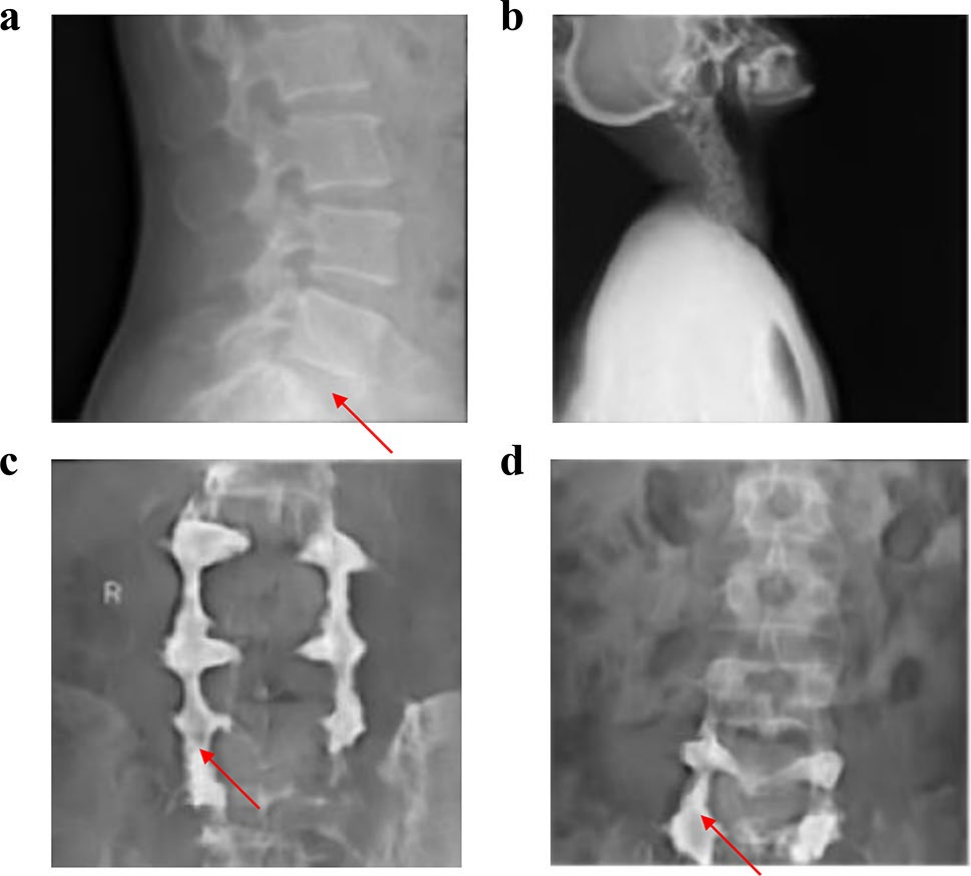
Selected examples from the proof-of-concept conditional generation of synthetic spine radiographs with a specific abnormality label options: No finding, Disc space narrowing, Foraminal stenosis, Osteophytes, Spondylolisthesis, Surgical implant, Vertebral collapse, Other lesions. (a) disc space narrowing, (b) no finding, (c–d) surgical implant.
